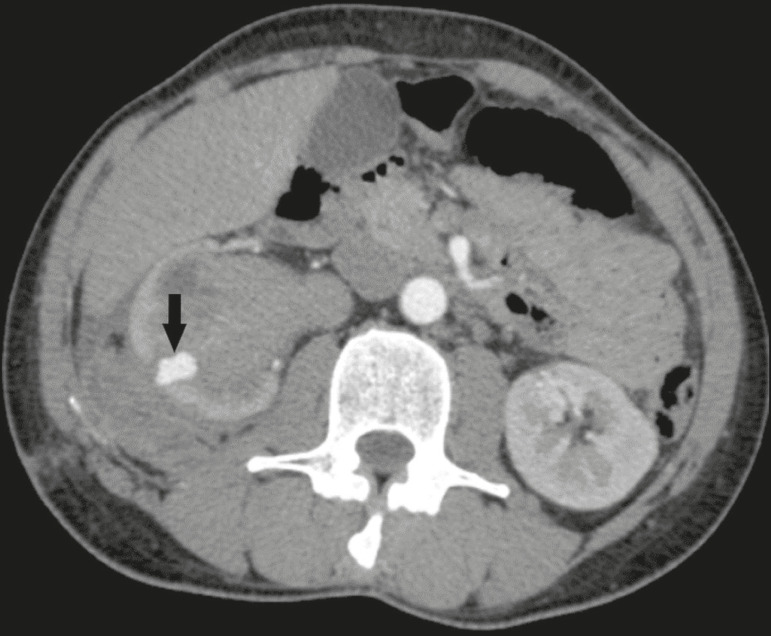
Axial CTA, in the corticomedullary phase, showing a pseudoaneurysm in the renal middle segment and a perirenal hematoma on the right.
